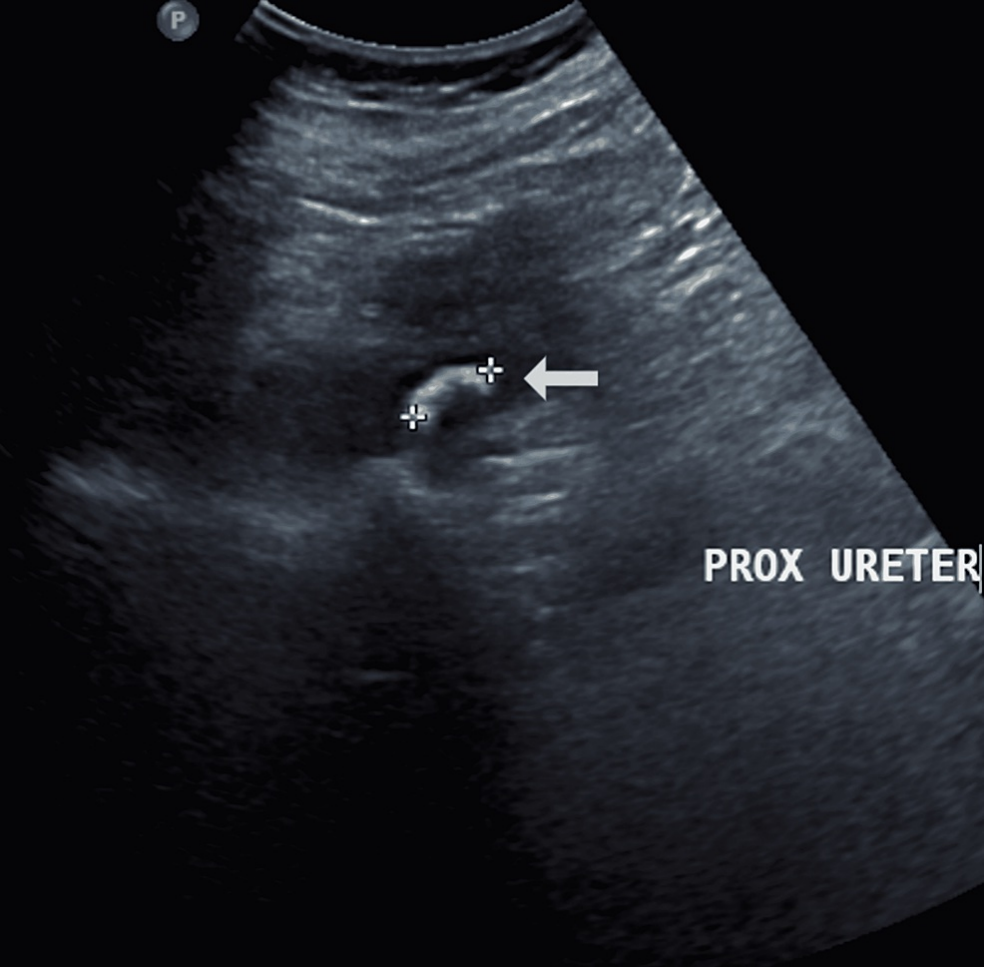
Ultrasonography of the abdomen showed a 15 mm calculus in the proximal right ureter at the pelvic-ureteric junction
